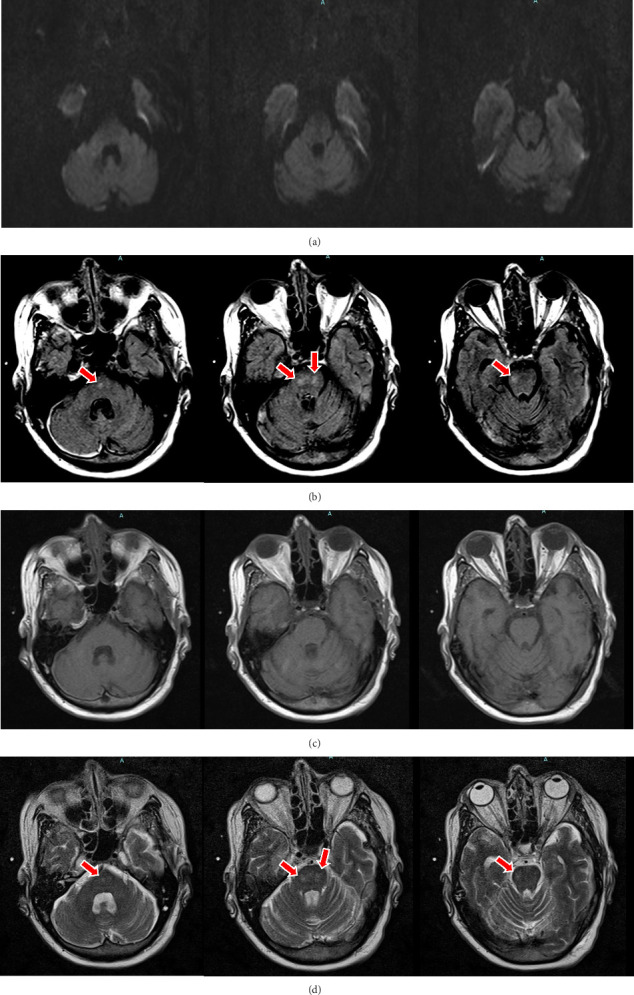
Magnetic resonance imaging brain. (a) The DWI images of the pons appear to be within the normal limit. (b) FLAIR images reveal patchy hyperintensities within the pons. (c) The T1 images appear to be within the normal limit. (d) The T2 images again reveal a hyperintensity within the pons.
